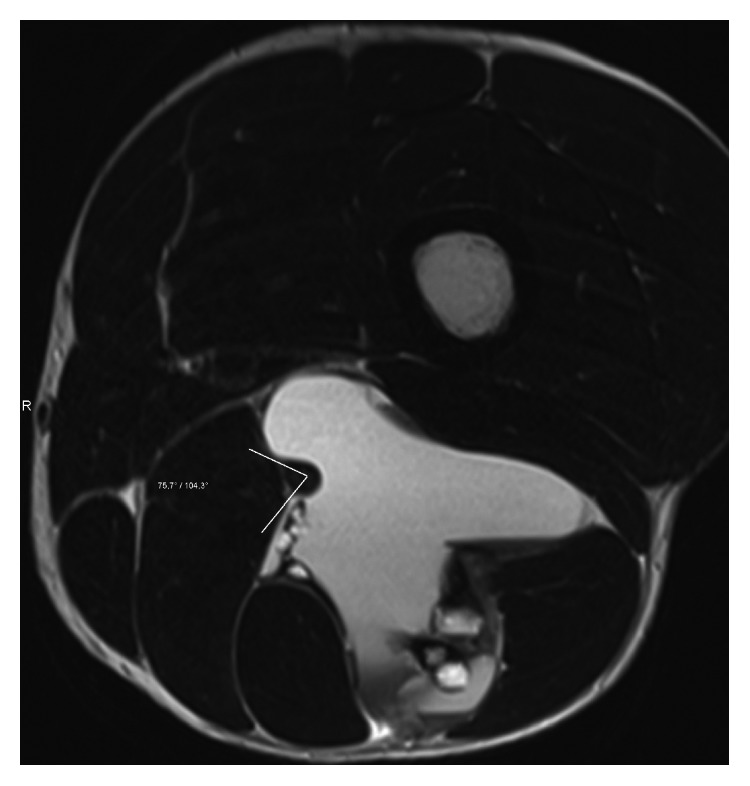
Preoperative MRI scan in a patient with type A sciatic nerve involvement and G3 pleomorphic sarcoma.
